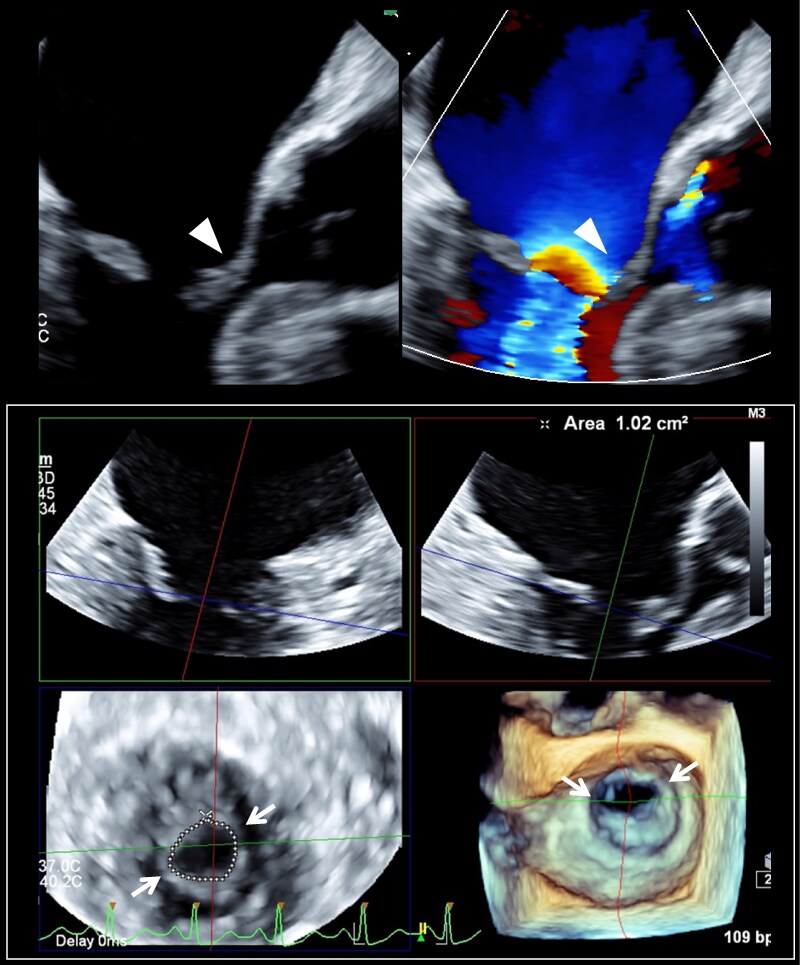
Transoesophageal echocardiography during hospitalization for congestive heart failure in November 2023 demonstrated severe mitral valve stenosis. The mitral valve leaflets showed marked thickening with doming of the anterior leaflet (arrowhead), and commissural fusion (white arrow) with severe restriction of valve opening was evident. The mitral valve area was calculated as 1.02 cm² using three-dimensional multiplanar reconstruction analysis.
